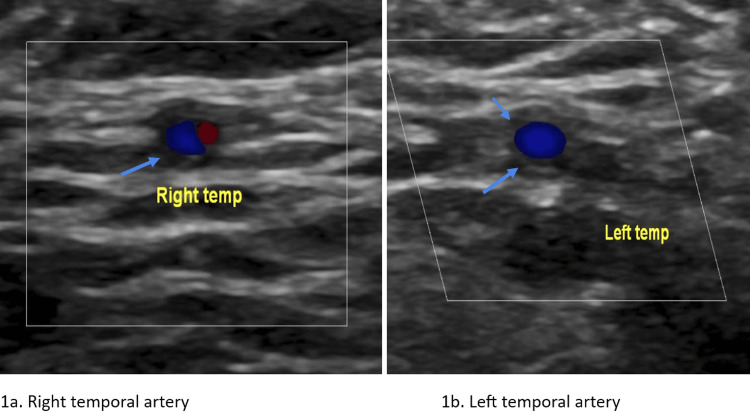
Temporal artery doppler (right and left) showing halo sign
